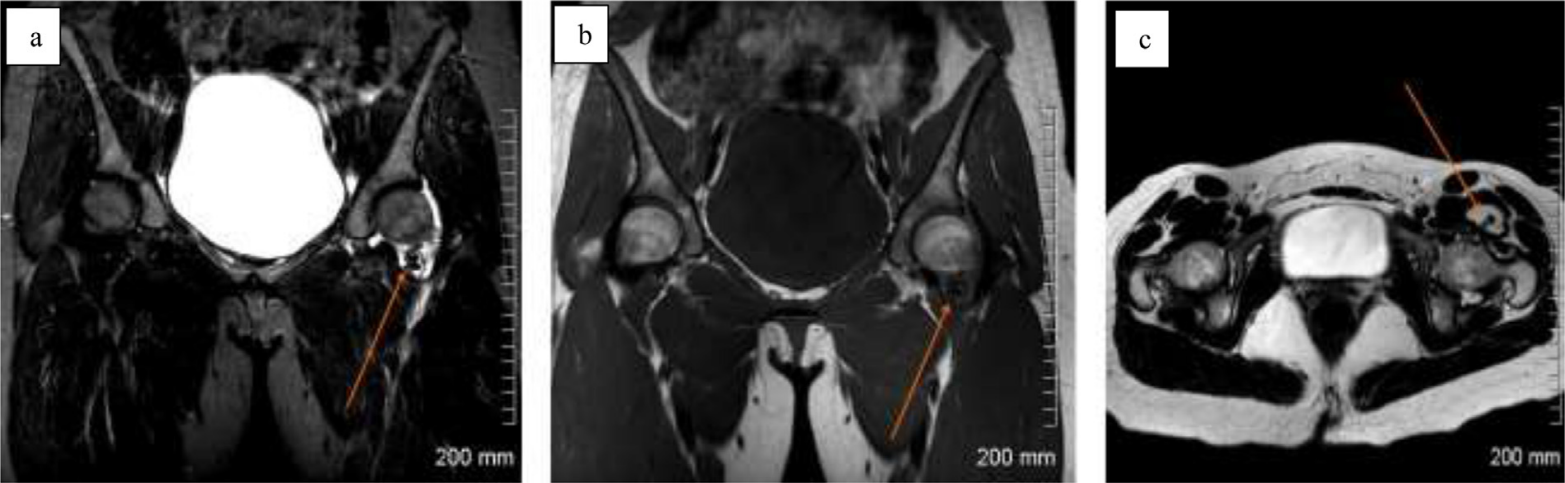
Case 1. Pelvic MRI sequences in a 15-year-old female. MRI demonstrates a moderate left hip effusion of intermediate T1 signal, implying hemosiderin content, with hypointense filling defects in the anterior recess of the joint. (a) Coronal short tau inversion recovery (STiR) sequence of the pelvis with left hip effusion (arrow), (b) coronal T1 sequence with left hip effusion (arrow), (c) axial T2 sequence of the pelvis with left hip effusion (arrow).
